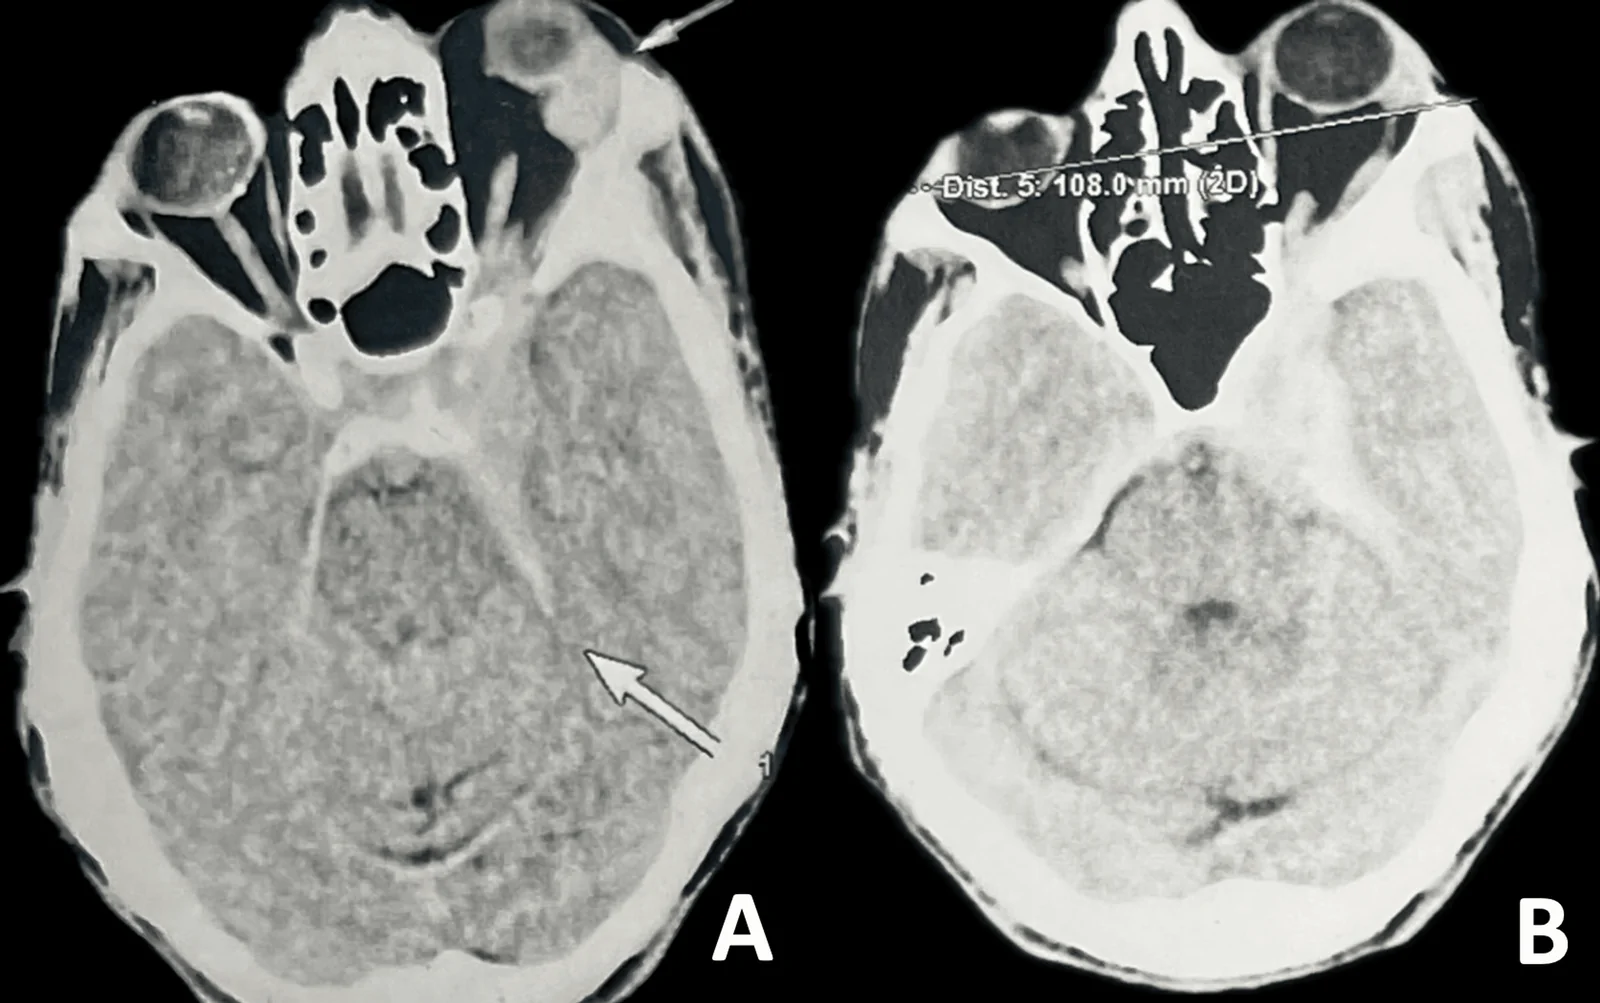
Brain CT scan with and without contrast enhancement (axial views) (A) Pre-contrast axial CT: A well-defined, rounded, isodense lesion is identified within the left orbit, exerting mass effect on adjacent orbital structures (thin arrow). A second isodense lesion with irregular margins is noted extending posteriorly from the orbital apex to the lateral wall of the cavernous sinus, with involvement of the left tentorial leaflet (thick arrow). (B) Post-contrast axial CT: Both lesions demonstrate avid and homogeneous contrast enhancement, consistent with highly vascularized or inflammatory pathology. Measurement along the external bicanthal line confirms grade III proptosis.
